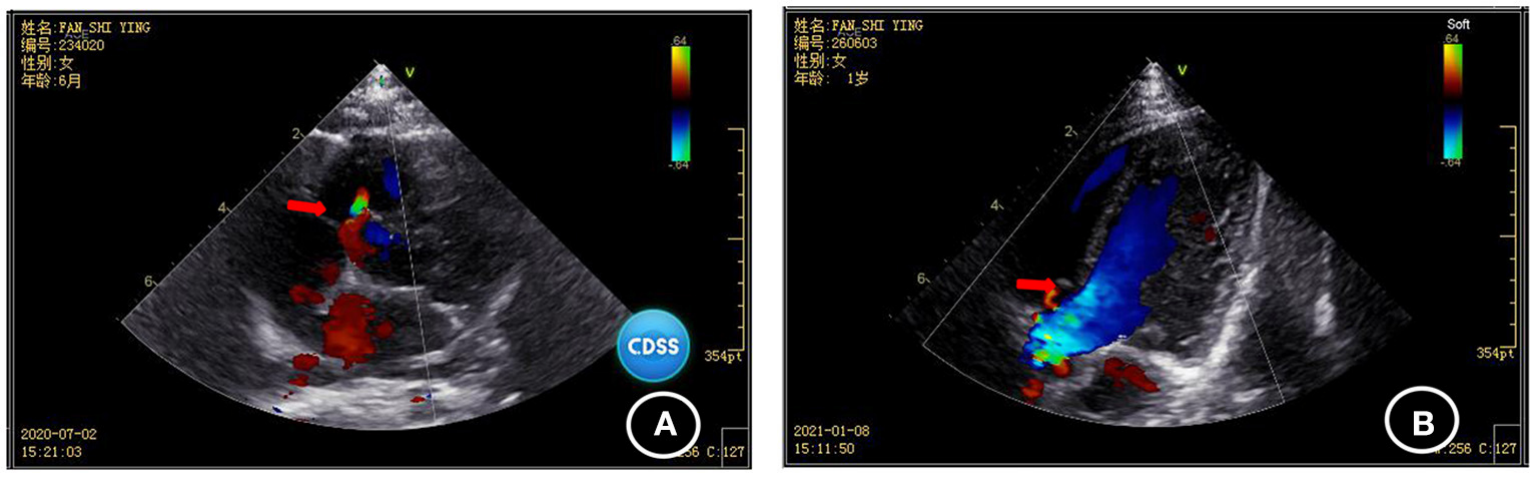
Comparison of the results of two cardiac ultrasonographies of the child (A) ventricular septal defect and pseudomonal tumor formation (rupture of about 2.5 mm and 1.8 mm) were found in July 2020; (B) Ventricular septal defect and pseudo membrane tumor formation (1.3 mm rupture) were found by heart color doppler ultrasound in January 2021.
